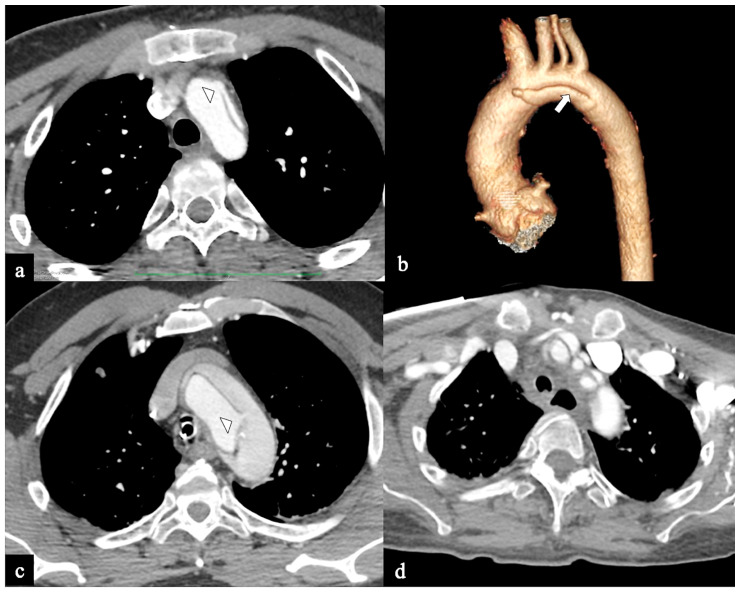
CTA images of non-A non-B dissection with Arch entry and isolated arch involvement or Configuration 2 (Arch alone). A 53-year-old man admitted to the hospital because of severe chest pain. (a) Axial CTA image displays the entry tear (indicated by the arrowhead) in the arch convexity. (b) A 3D-VR parasagittal reconstruction exhibits a localized arch dissection (arrow) without involvement of the supra-aortic trunks, along with the left vertebral artery originating from the arch (4-vessels arch). The patient received medical treatment. Subsequent CTA examinations conducted at three, six, twelve months, and two years in the follow-up period revealed no progression of the aortic abnormality. (c) Axial CTA image shows primary entry tear (arrowhead) in the arch in a 70-year-old man with acute chest pain and stroke. (d) In the same patient, a more cranial axial CTA image shows supra-aortic vessel dissection involvement.
